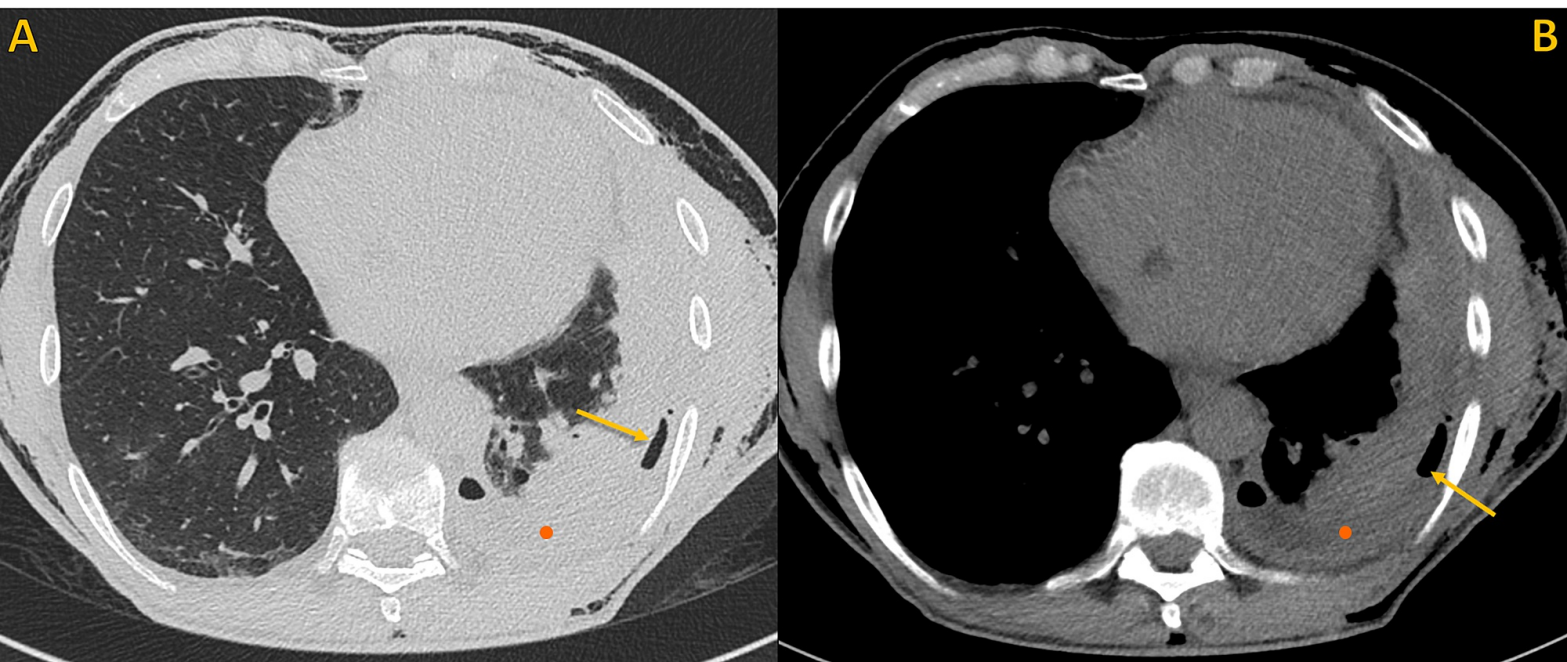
Non-contrast chest CT, same axial plane Non-contrast chest CT, same axial plane. A) Lung window and B) Soft tissue window, showing reexpansion of the left lung base, smaller left pleural effusion (orange circle), and a minimal pneumothorax (yellow arrow).
